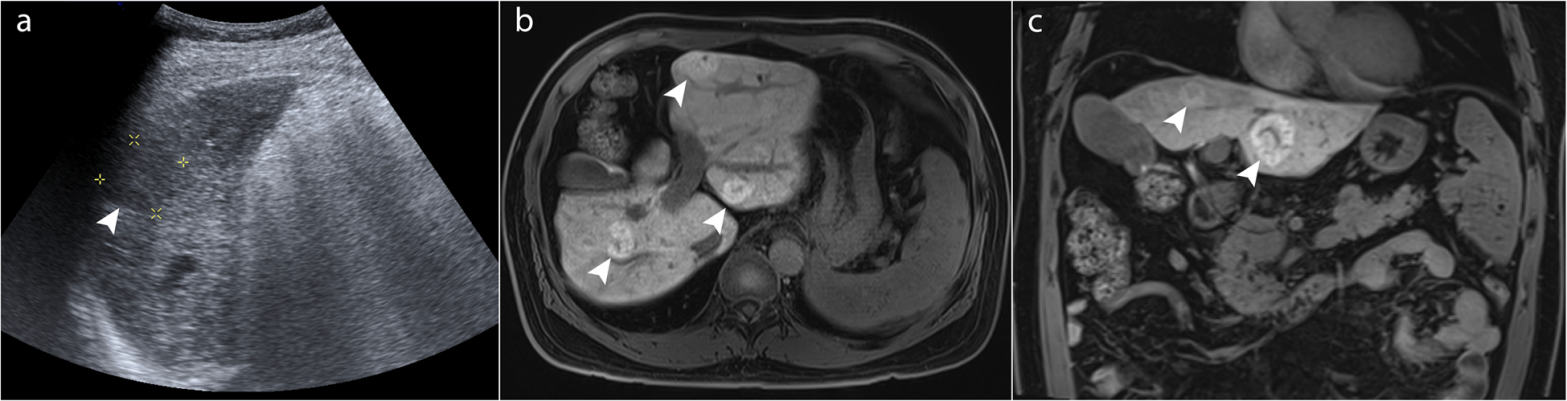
A 59-year-old patient with chronic hepatitis B and cirrhosis who has at least 10 hypoechoic lesions on US (a) measuring 2–3 cm (arrowhead). On gadoxetic acid-enhanced magnetic resonance imaging (b, c), these are shown to be focal nodular hyperplasia (FNH)-like nodules in cirrhosis (arrowheads). This patient cannot be reliably screened with ultrasound. In fact, FNH-like nodules are identical to classic FNH and are benign. They occur in cirrhosis and are believed to originate from acquired hyperplastic responses to vascular alterations associated with cirrhosis
